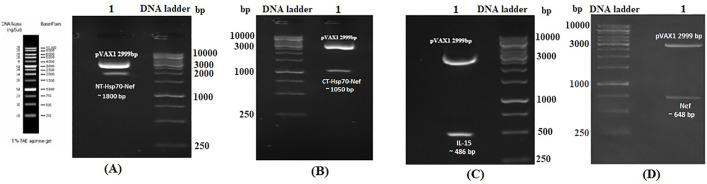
Validation of recombinant plasmid constructs via restriction digestion. A) Digestion of pVAX-NT-Hsp70-Nef with *Bam*HI and *Xho*I; B) pVAX-CT-Hsp70-Nef digested with the same enzyme set; C) Verification of pVAX-IL-15 through *Nhe*I and *Pst*I digestion; D) Digestion of pVAX-Nef using *Nhe*I and *Bam*HI. A 1 kb DNA ladder (Fermentas) was used as a molecular size marker.
